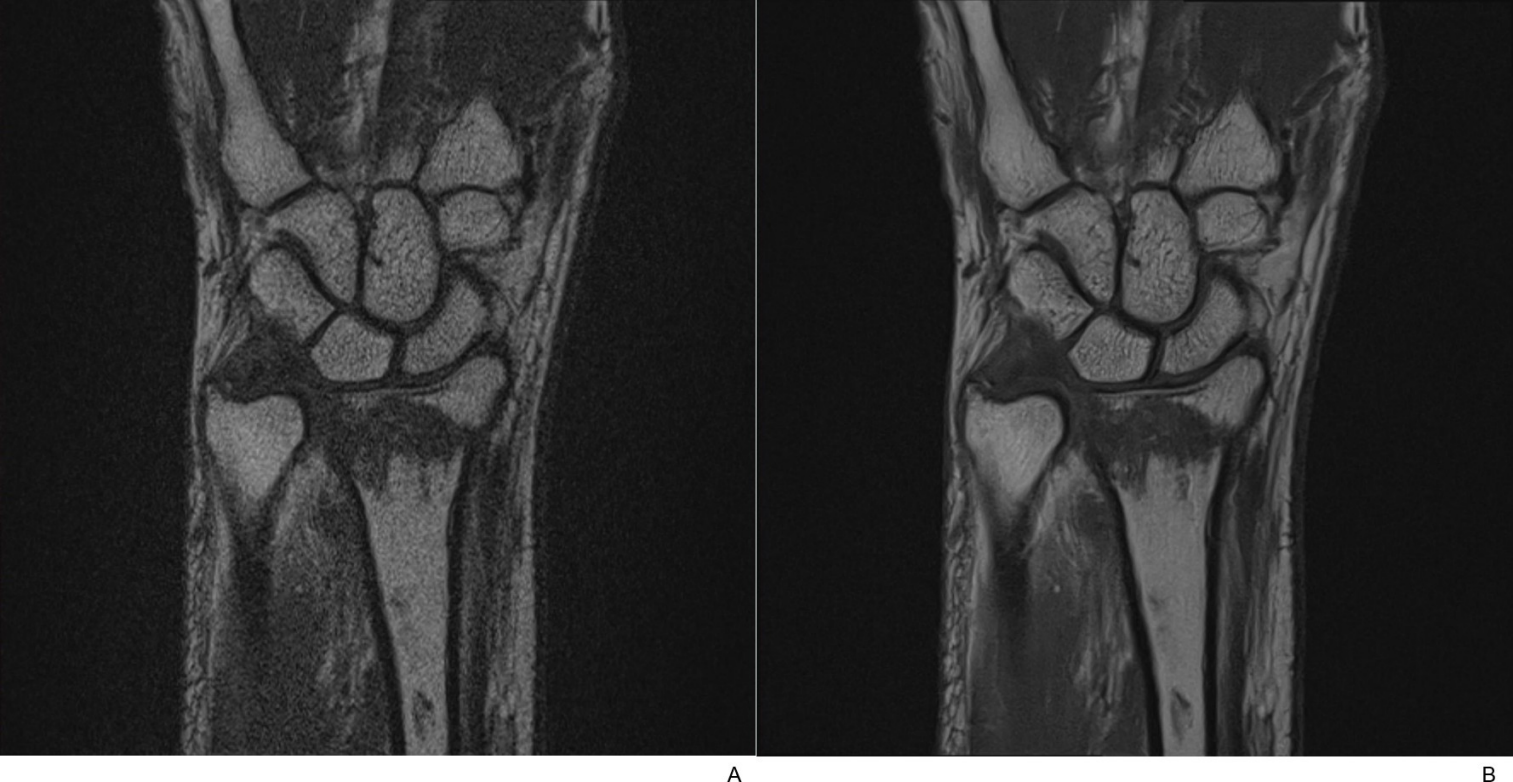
A 57-year-old female with a distal radius fracture. (A) Coronal T1-weighted TSE_S_ image and (B) the corresponding TSE_DL_ image. TSE_DL_ images showed superior rSNR, rCNR and rCR compared to TSE_S_ images.
